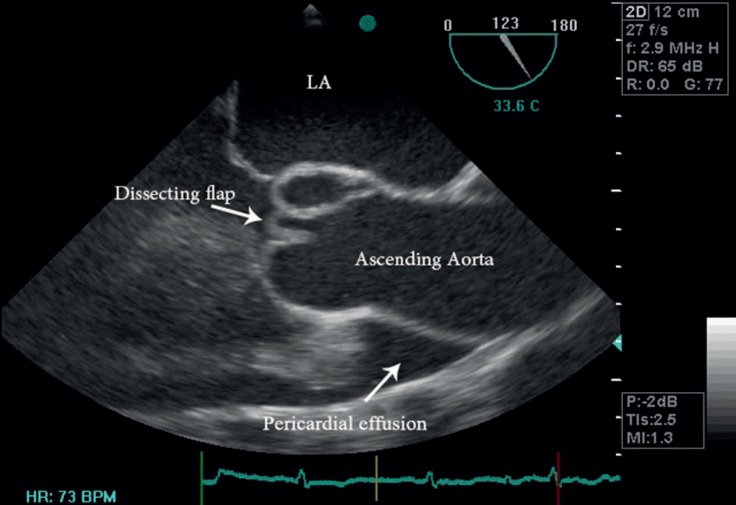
Transesophageal echocardiography in the mid-esophageal view, demonstrating a linear dissection flap originating from the non-coronary sinus of Valsalva
